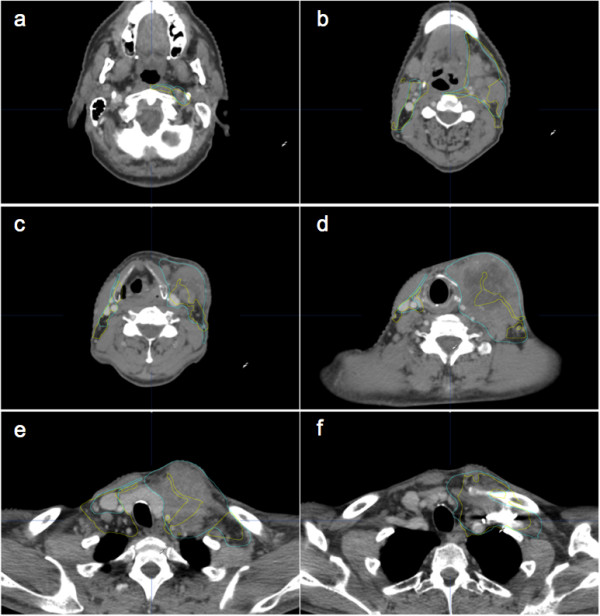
Selected CT slices of patient 16 (N3-stage) with automatic (yellow lines) and manual (blue lines) segmentations. Large normal anatomy distortions on the left side of the patient fool the algorithm that generates inaccurate automatic segmentation (images c, d, e). Levels depicted in the different panels: a = RS and RP left; b = 2 bilaterally, 1b and RP; c and d = 3 bilaterally; e = 4 bilaterally; f = RCL left.
